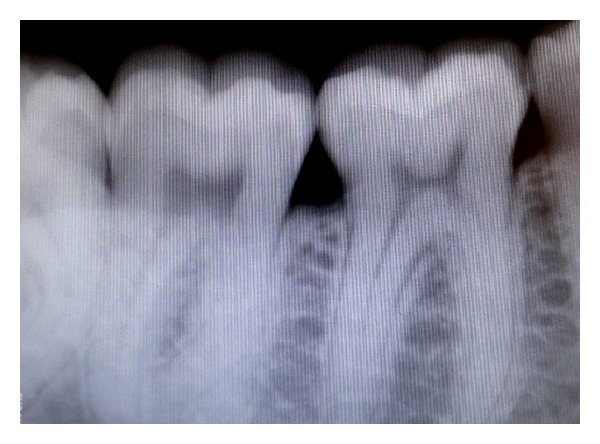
Periapical radiograph showing mild crestal bone loss between first and second molars.
